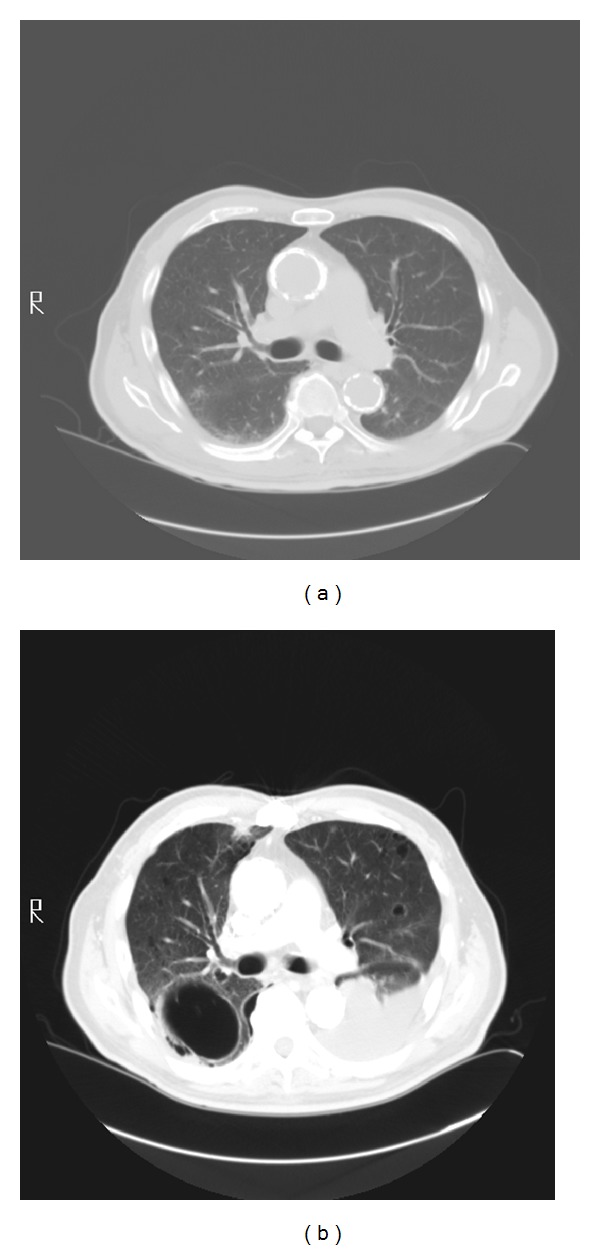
CT scans of the chest at 6-month interval showing a new left pleural effusion and a large thin-walled cystic lesion in the right lung.
